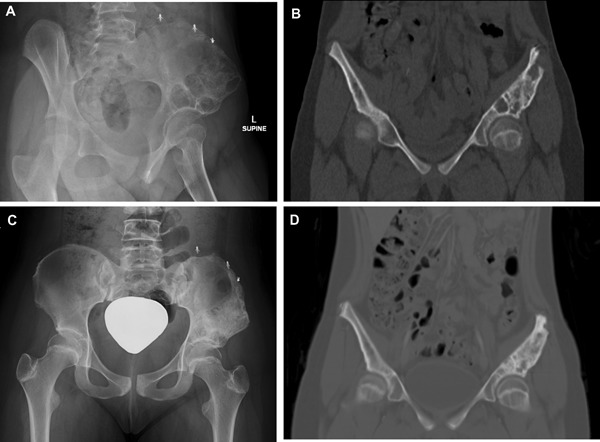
Patient with aneurysmal bone cyst of pelvis. (*A*, *B*) Judet plain film and sagittal CT before denosumab therapy and after two prior surgical interventions (*C*, *D*) and posteroanterior pelvis plain film and sagittal CT after denosumab therapy.
